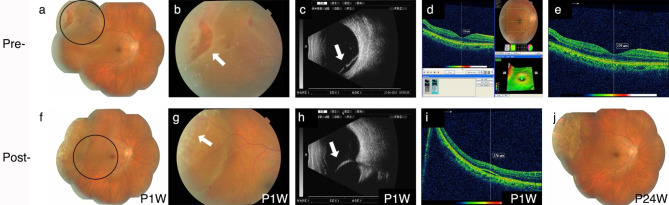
FCB scleral buckling efficacy in the pseudophakic eye of patient 4 (a) Fundus image showing retinal detachment pre-surgery. (b) The amplified circle area from A shows the detached retina (white arrow). c–d. OCT shows an affected and detached macula. e. Fundus image of FCB scleral buckling at 1-week post-surgery (P1W). f. Fundus image showing clear and distinct photocoagulation spots (white arrow). g. Post-operative B-ultrasound scan results showing the FCB’s apparent pressure on the sclera (white arrow). h. OCT was showing the reattached macular. i. Fundus image at 24 weeks post-operation (P24W) showing the well-reattached retina
